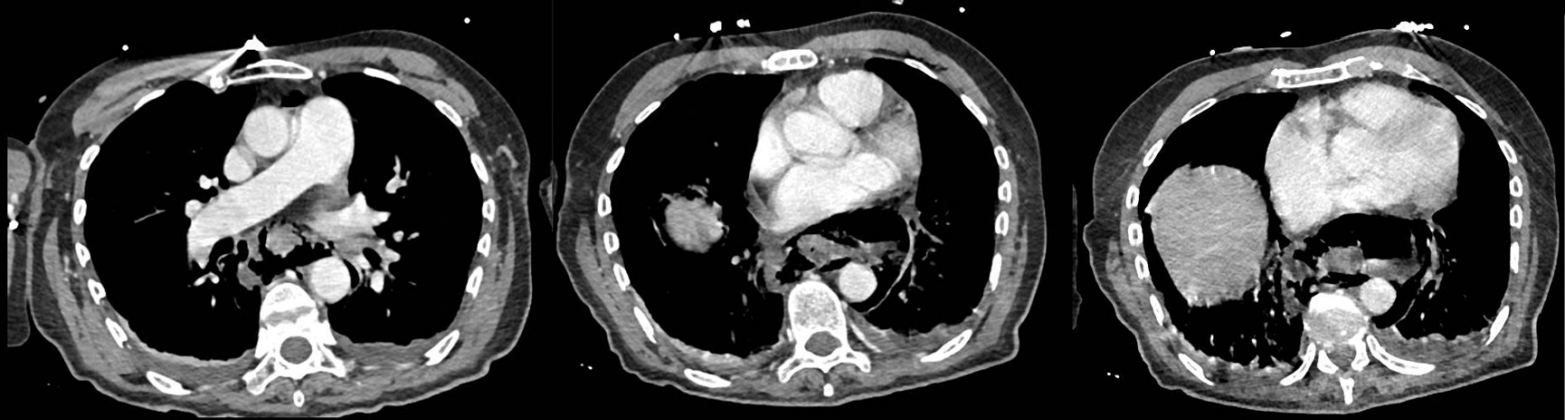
Contrast‑enhanced chest CT. Axial views in the parenchymal window show intramural hematoma, peri‑esophageal air collections indicating esophageal perforation, esophageal wall thickening, and bilateral pleural effusion. Source: Charleroi University Hospital.
